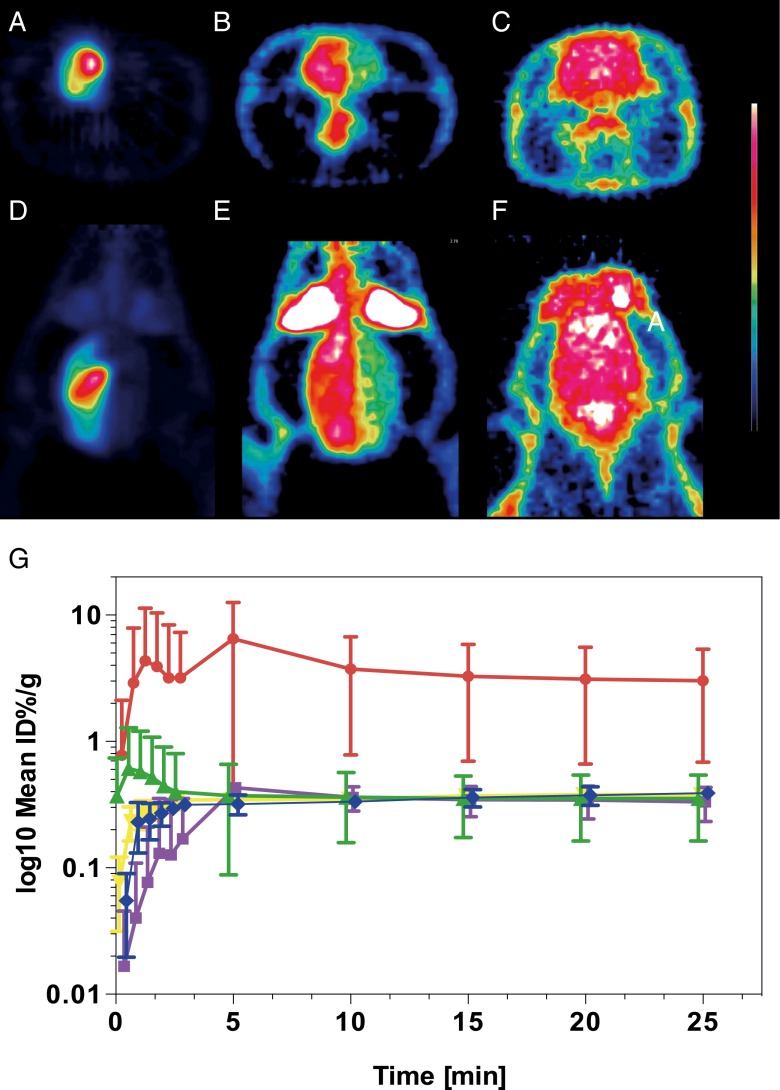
Distribution and changes in tissue radioactivity of ^18^F-FDG following selective intra-arterial injections in the internal and common carotid arteries and systemic injections in the tail vein. ^18^F-FDG PET, transaxial and coronal planes, following: a, d injection in the left internal carotid artery (*iICA*); b, e injection in the common carotid (CCA); c, f injection in the tail vein. g Changes in tissue radioactivity over time expressed as percentage of the injected dose per gram tissue (%ID/g; *Y* axis = log_10_ mean %ID/g) following: injection in the iICA in the left (ICA LH, *red dot*) and the right (ICA RH, *purple square*) cerebral hemispheres; following injection in the left CCA in the left (CCA LH, *green triangle*) and the right (CCA RH, *yellow inverted triangle*) cerebral hemispheres; following i.v. injection (IV, *blue rhombus*). The differences in mean values at 25 min are significant between ICA LH and ICA RH (*p* = 0.009), ICA LH and CCA LH (*p* = 0.008) and between ICA LH and IV (*p* = 0.004). PET images are summed over 25 min. Error bars indicate ±1 SD
